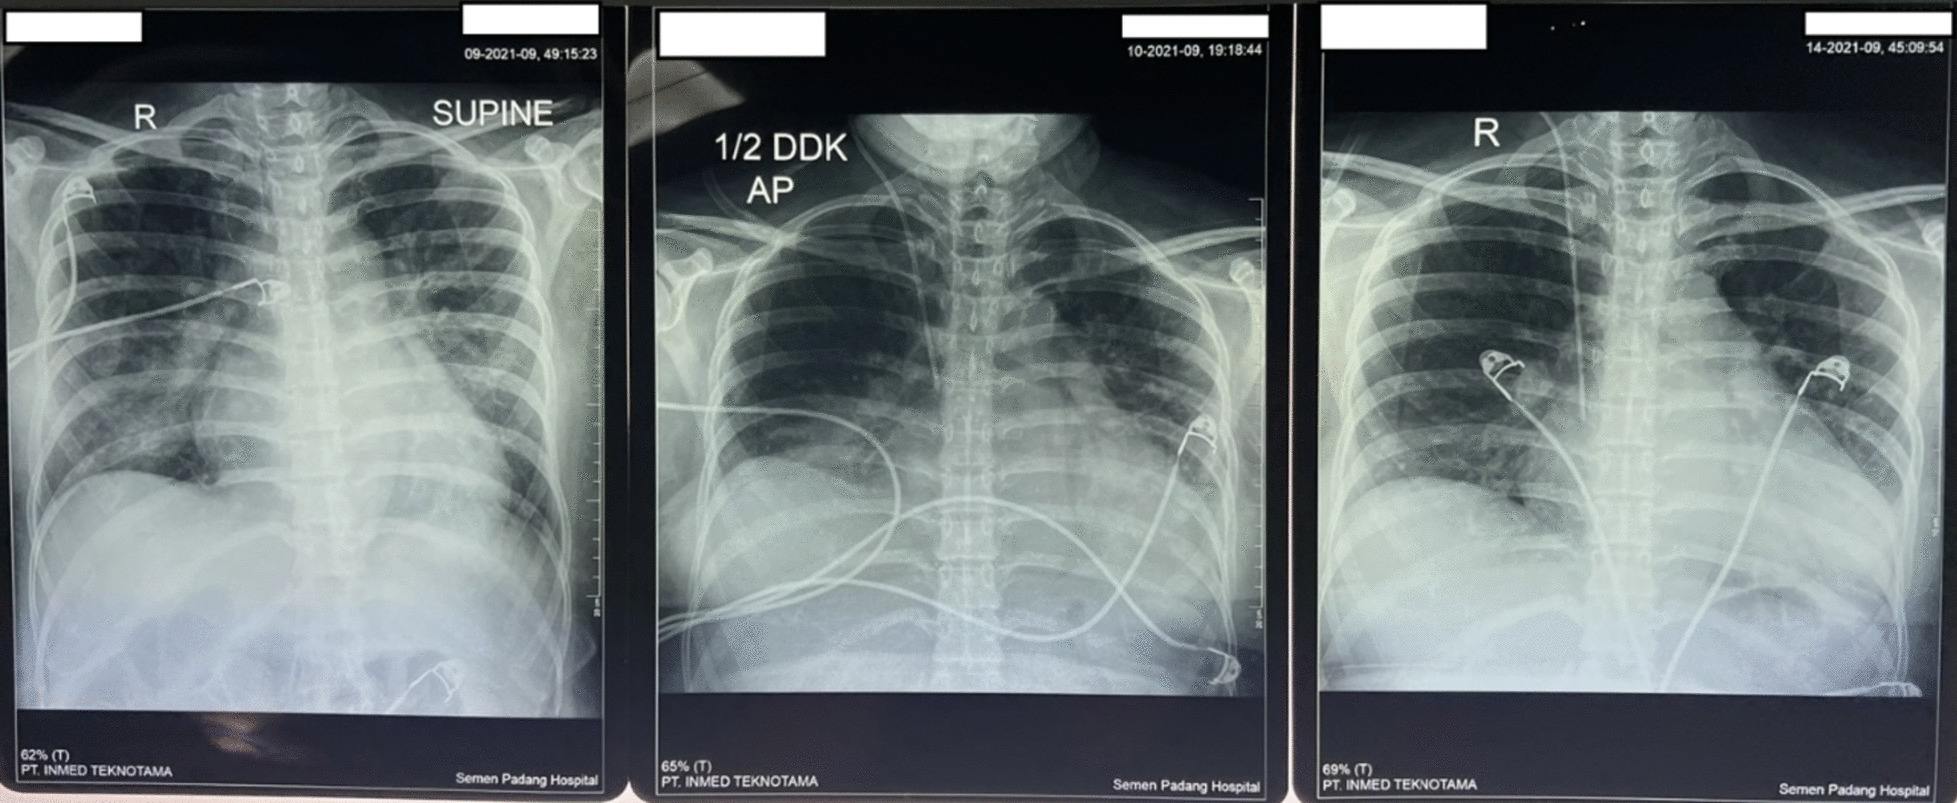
Chest X-ray examination (left: post operative day 0, middle: post operative day 1, right: post operative day 6)
